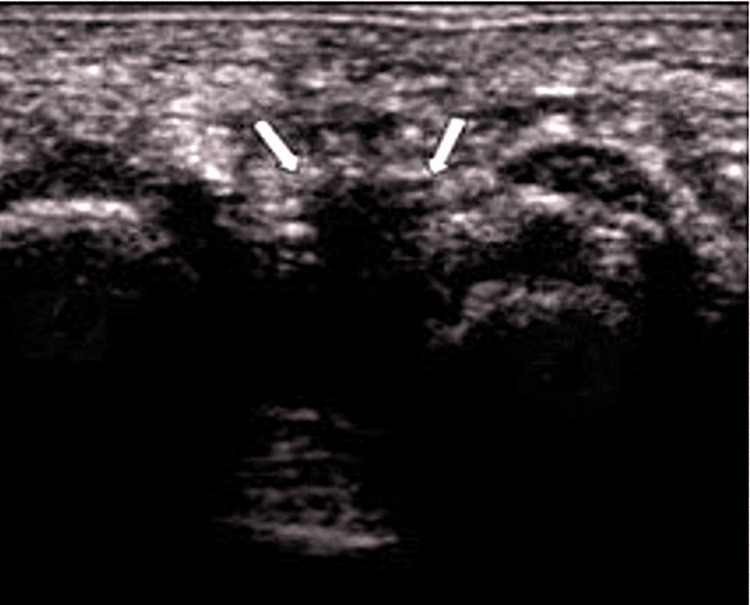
Ultrasound image showing Morton's neuroma in a patient. Image Credit: Manare Jaai The image presents a 7 mm hypoechoic ovoid lesion along the longitudinal axis between the third and fourth metatarsals. These findings are crucial for diagnosing the underlying conditions affecting the patient.
